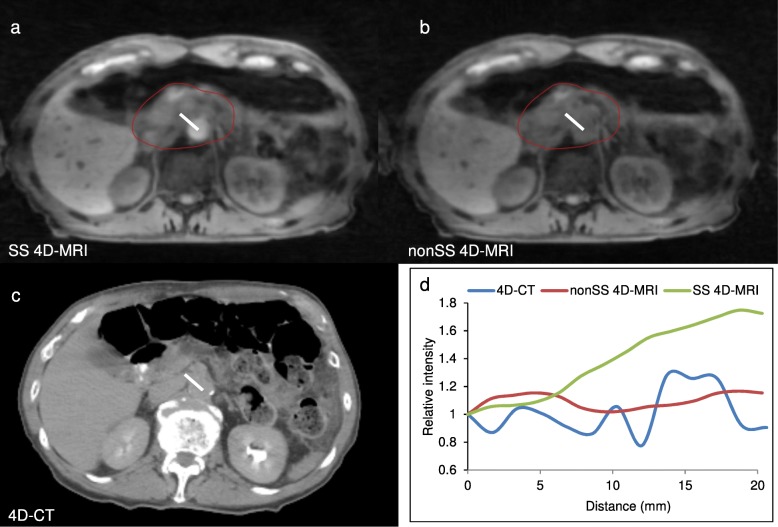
Example images from slab-selective 4D-MRI (a), non-slab-selective 4D-MRI (b) and 4D-CT (c) for the same patient. Red circle indicates the tumor region and green arrow indicates the involved blood vessel. d The relative image intensity profile across the tumor and aorta interface indicated by the white line on the 4D-CT image in (c), with slab-selective-4D-MRI showing higher contrast across the tumor/vessel interface than that non-slab-selective-4D-MRI and 4D-CT
